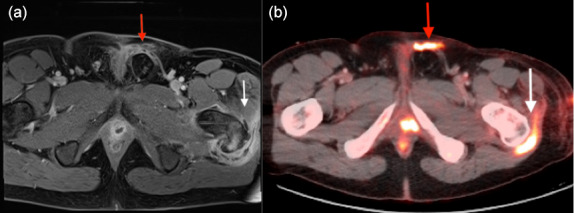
(a) MRI suggests osteomyelitis in the left greater trochanter (white arrow) and two small abscesses near the prostate with periurethral fluid infiltration (red arrow). (b) FDG PET/CT shows high metabolic activity at the trochanter (white arrow) and along the urethrocutaneous fistula's path (red arrow).
